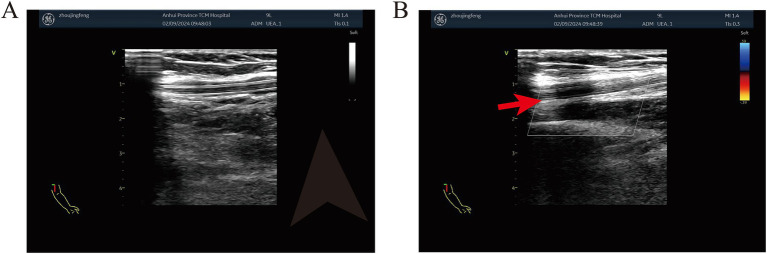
Duplex ultrasound findings of the left upper extremity. (A) Grayscale ultrasound image showing the axillary vein. (B) Color Doppler ultrasound demonstrating reduced or absent flow in the affected vein (arrow), consistent with thrombosis.
